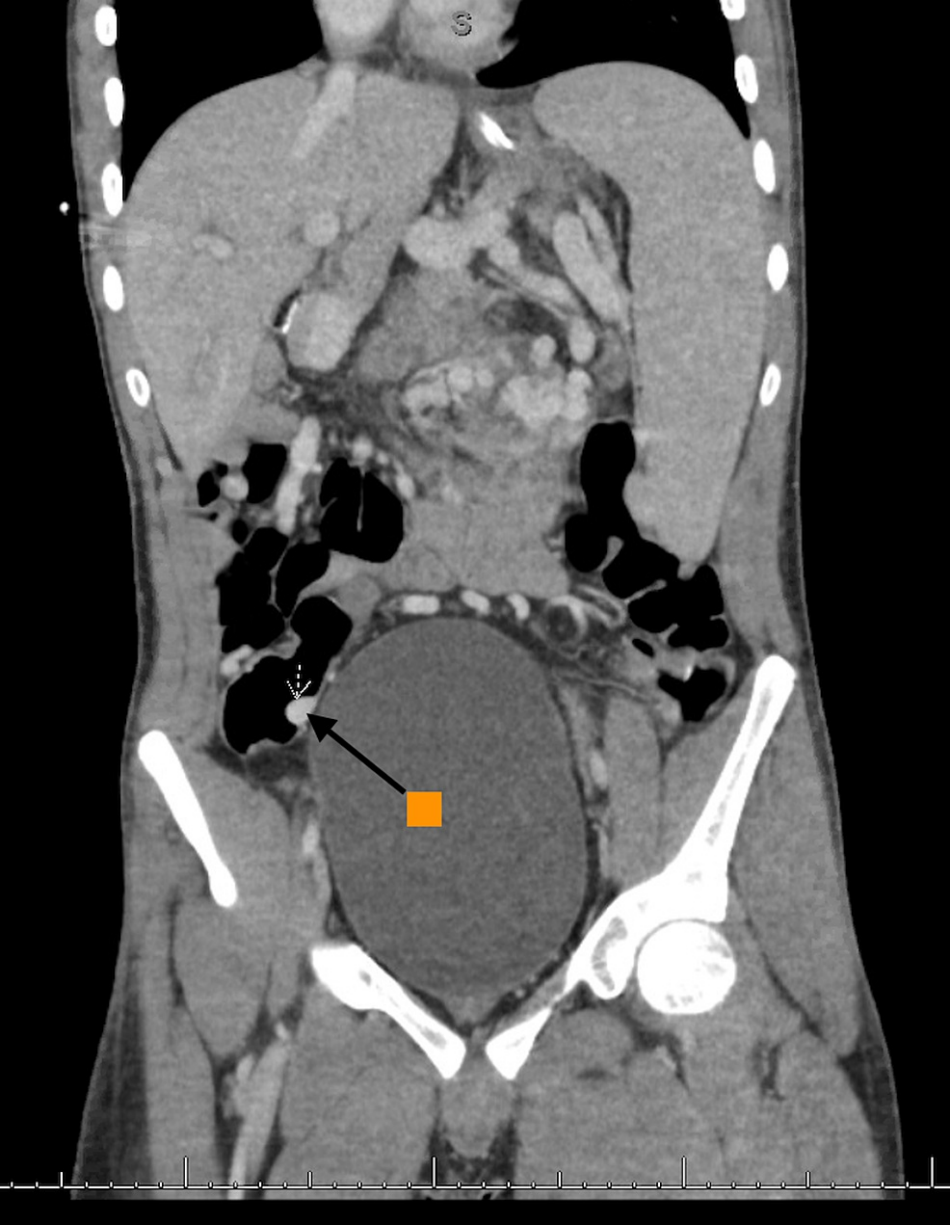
CT angiogram of the abdomen showing small second cluster of cecal varices (orange arrow) CT: computed tomography
